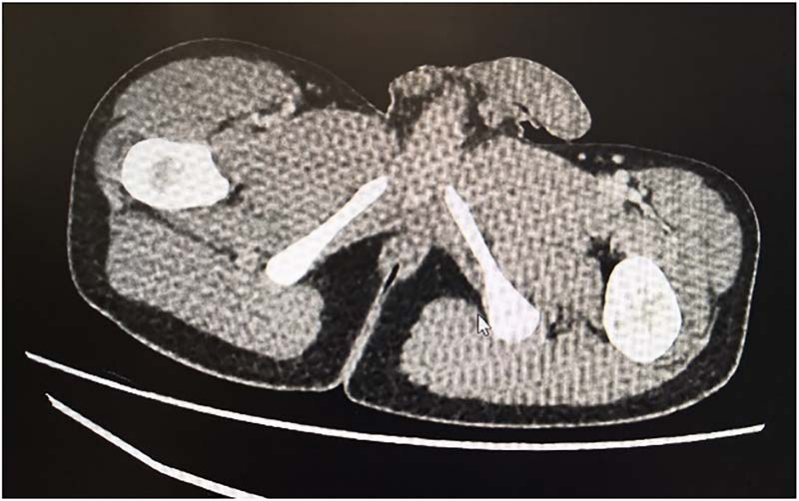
CT demonstrating resolution of the right gluteus maximus oedema.
